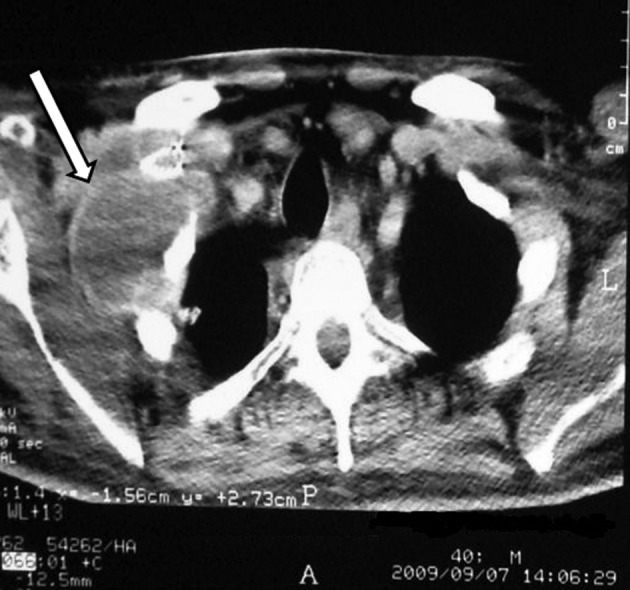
Visualization of the cystic transformation of the mass (arrow) with a contrast-enhanced computed tomography (CT) scan immediately after the repeat radiofrequency ablation (RFA) with the patient in supine position.
